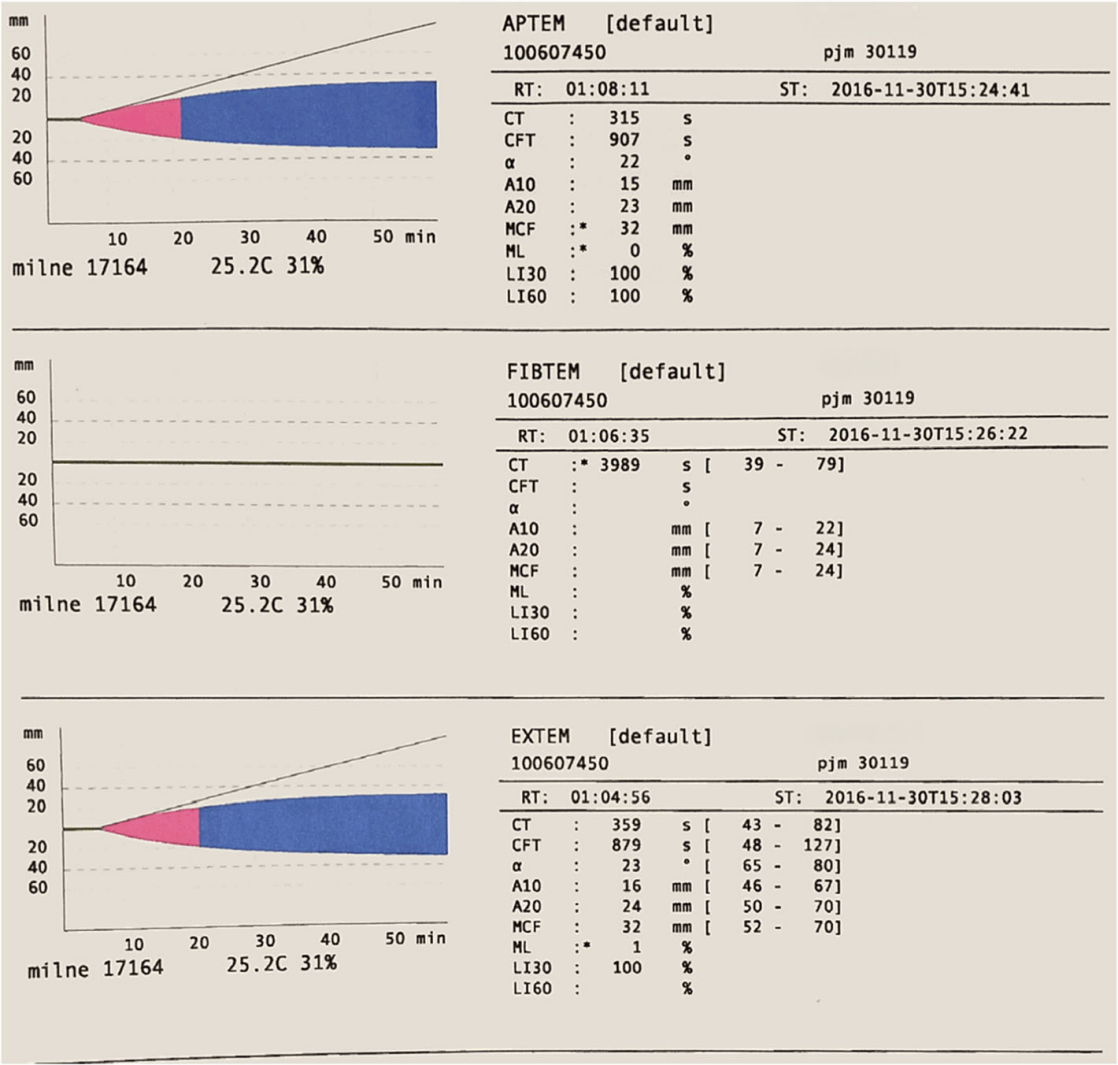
ROTEM® 1: ROTEM® results show very low amplitude fibrinogen; FIBTEM A10 is undetectable. EXTEM results show prolonged CT (359 s) and low clot A10 in the EXTEM (16 mm)
